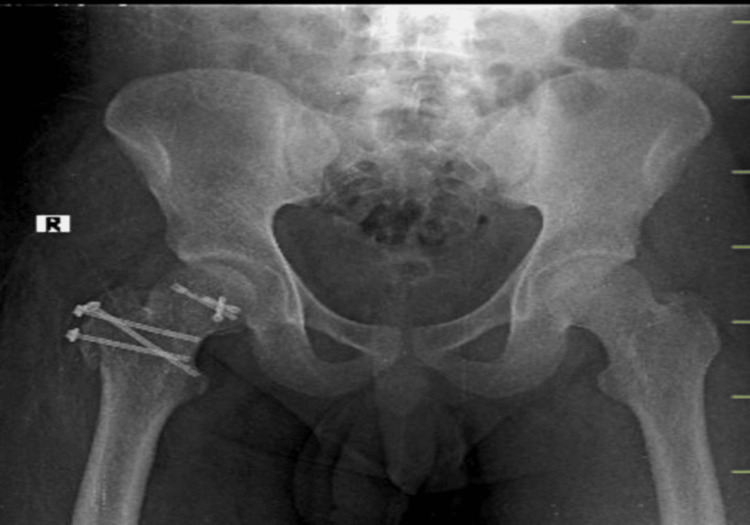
Four-week post-operative follow up anteroposterior radiograph of the pelvis with both hips. Four-week post-operative anteroposterior radiograph of the pelvis with both hips, demonstrating a well-maintained, concentric right femoral head with no screw penetration of the articular surface or joint space, and a symmetrical contralateral hip for comparison.
